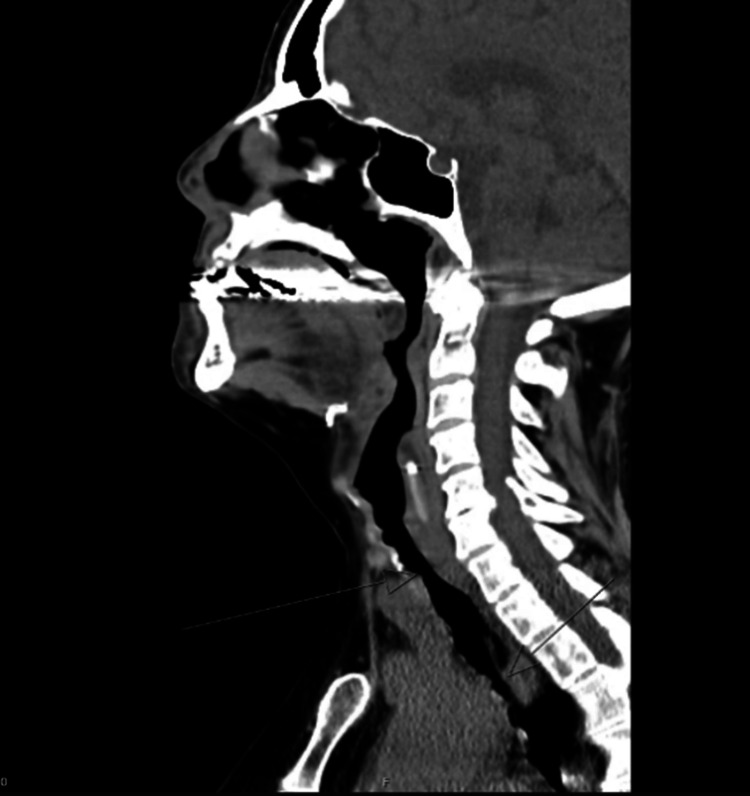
CT image of soft tissue neck without contrast (sagittal view) The image is showing multifocal areas of mucosal thickening in the subglottic region and in the trachea.
